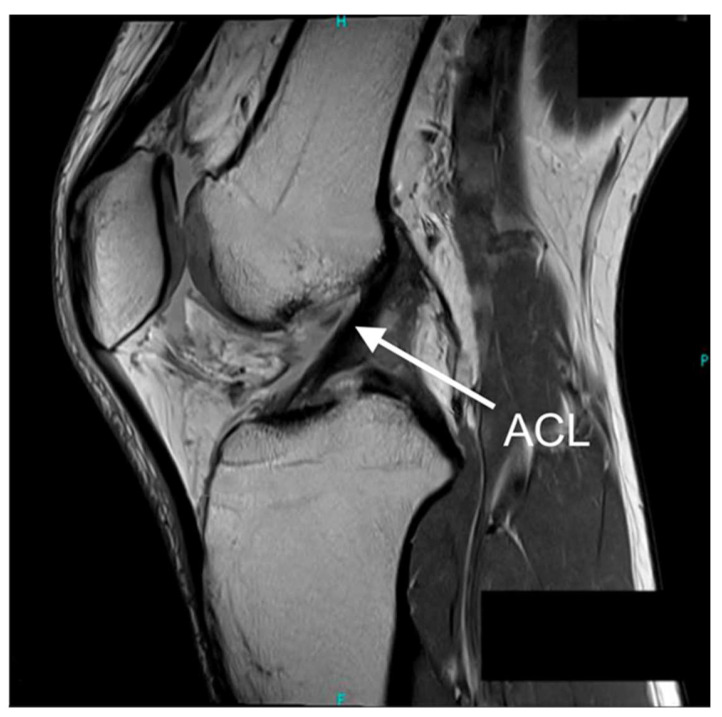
Sagittal view MRI (magnetic resonance imaging) scan of the knee joint. Intact ACL indicated by the arrow, demonstrating normal ligament continuity and positioning. Orientation labels: H—head (superior), F—foot (inferior), P—posterior. Adapted from [3], CC BY 4.0. Changes: original box annotation and panel label removed.
